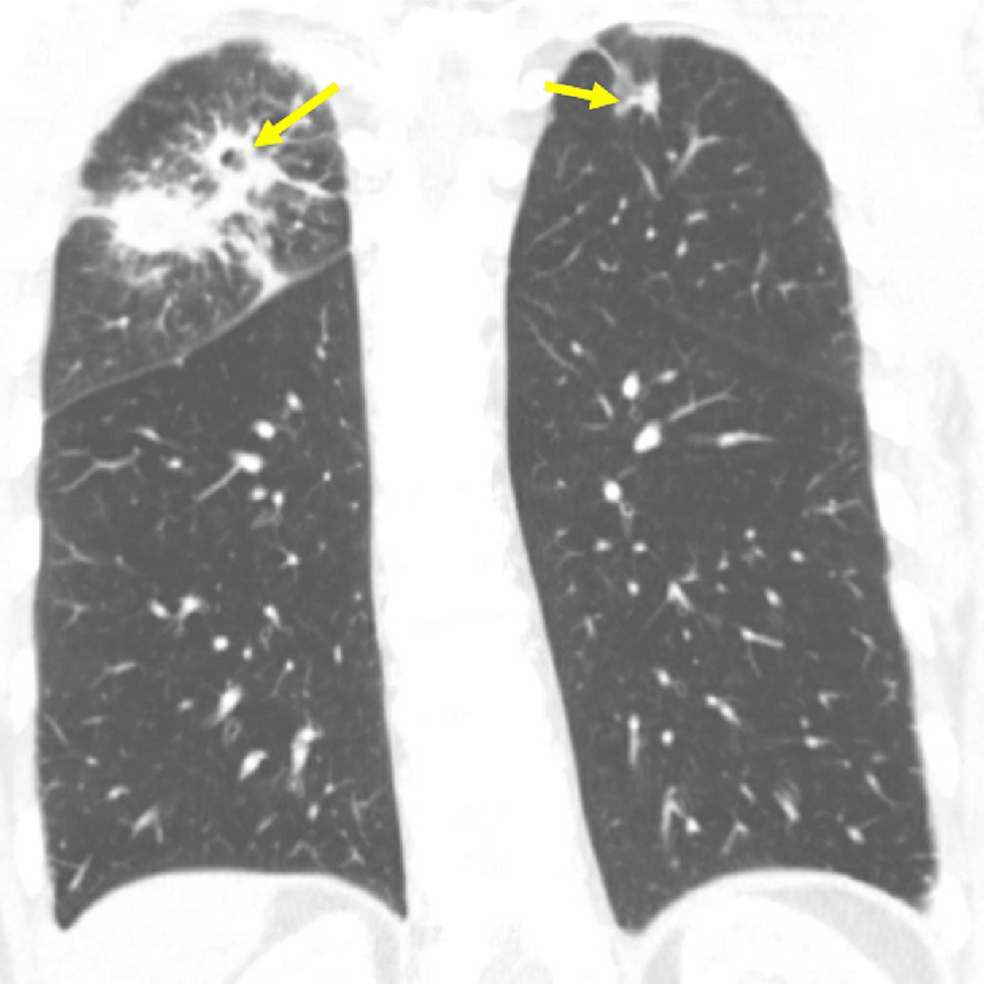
Chest CT showing right upper lobe multinodular patchy consolidation with mild cavitation and left upper lobe nodular consolidation (yellow arrows). CT: computed tomography
